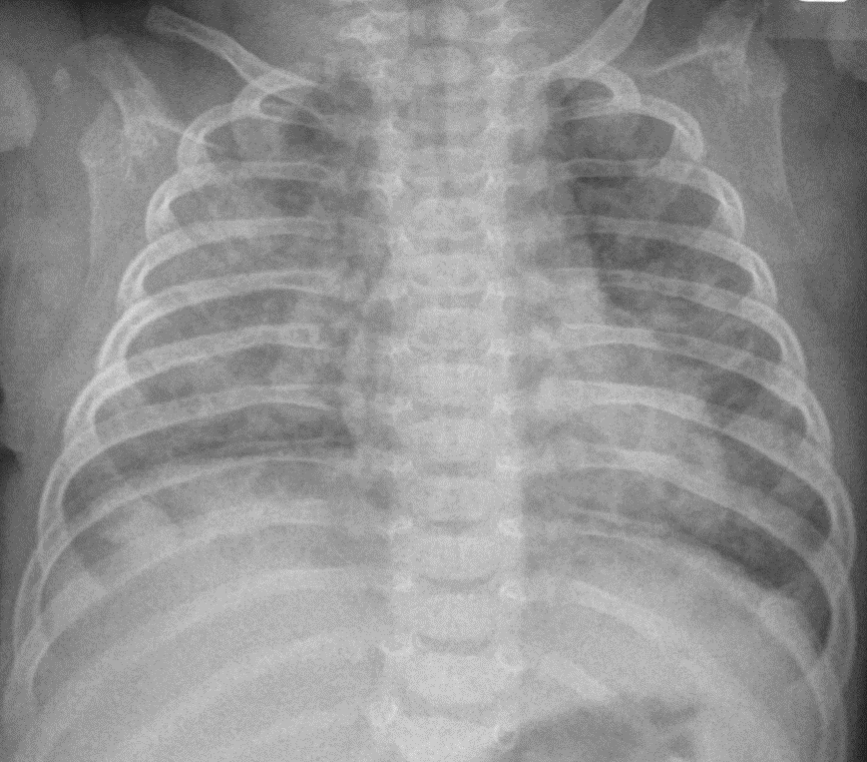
AP chest radiograph at the age of 7 months, before discharge home from the hospital. Generalized diffuse, hazy opacities and coarse interstitial markings with a small degree of hyperinflation – signs of bronchopulmonary dysplasia (BPD). Fibrin glue clot disappeared.
